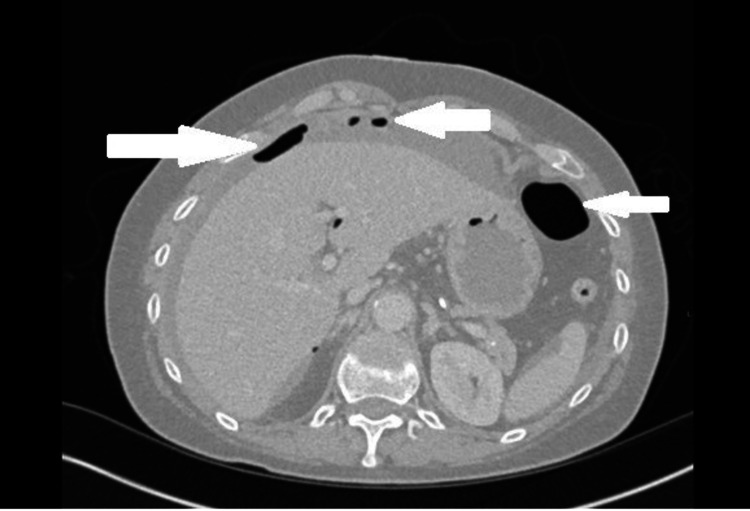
CT lung window helps to depict intra-abdominal air more than soft tissue window (see white arrows).
